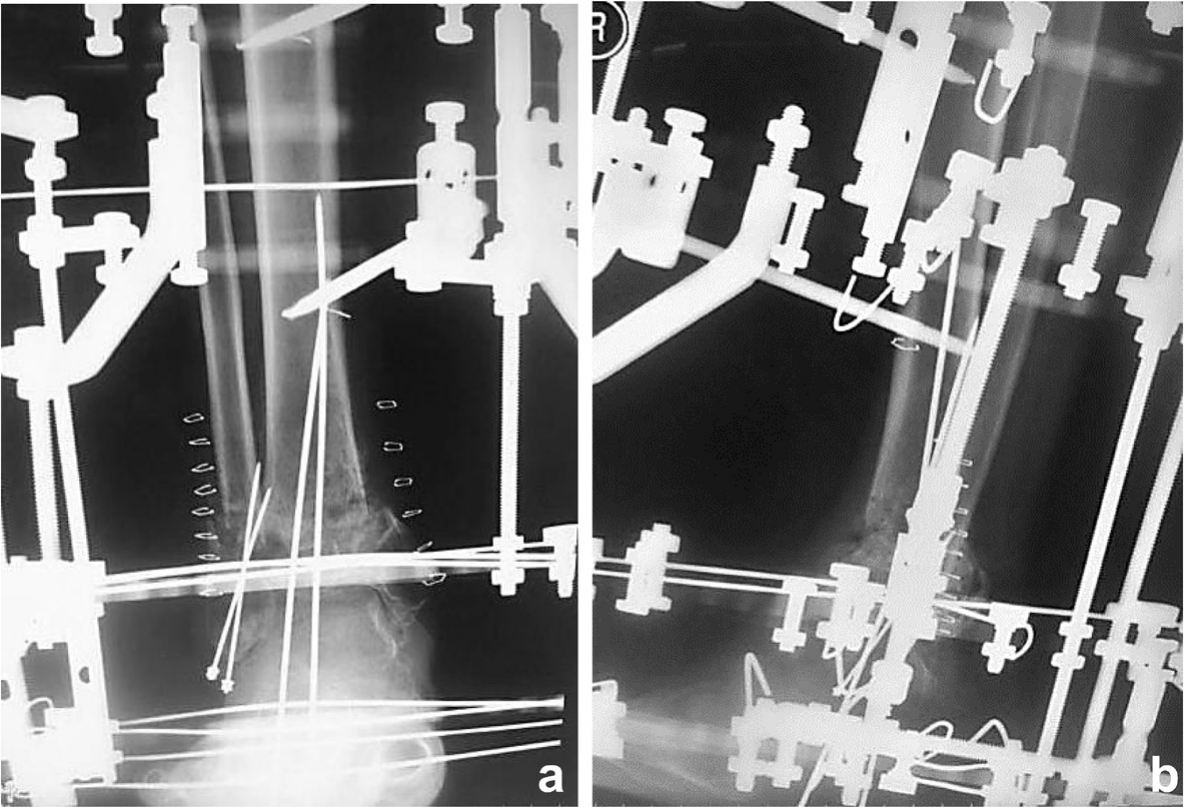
Postoperative radiographs. (a, b) Anteroposterior- and lateral-view plain radiographs after surgery, showing anatomical reduction.
